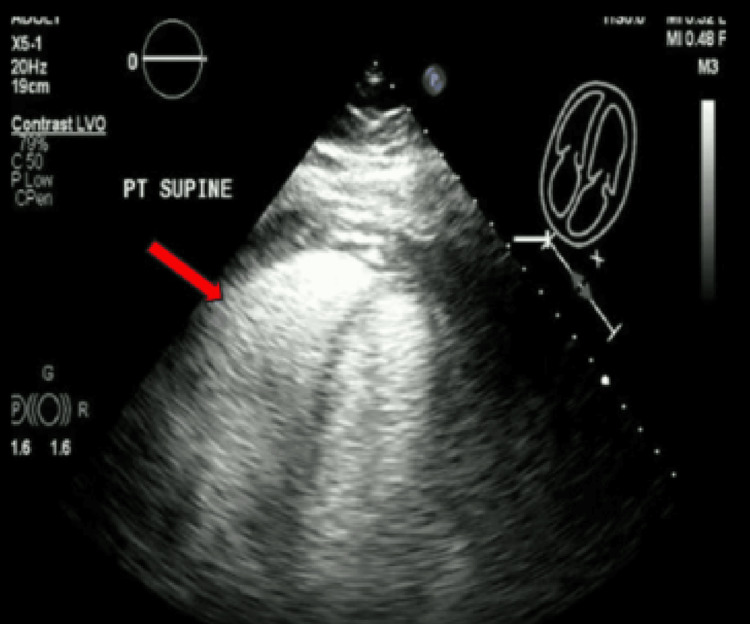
Transthoracic echocardiogram with contrast, 4-chamber view Transthoracic echocardiogram with contrast demonstrated a moderately dilated right ventricle with a preserved left ventricular size.
